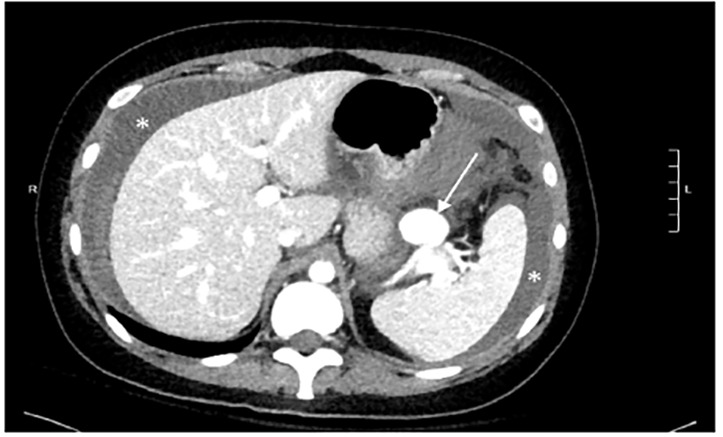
Computed tomography axial section showing a large splenic artery aneurysm (arrow). There is also significant hemoperitoneum surrounding the liver and spleen (asterisks).
